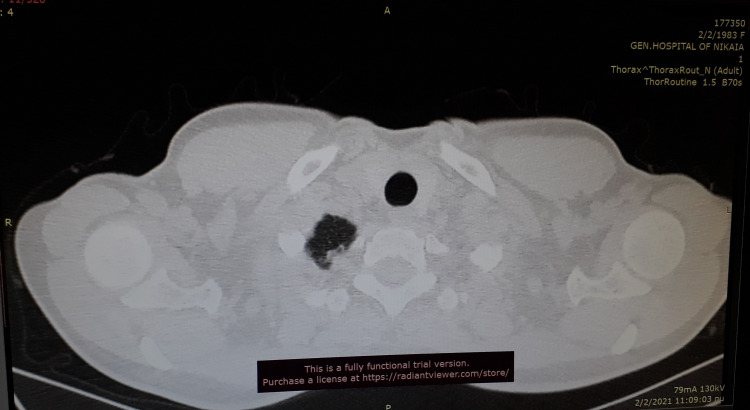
The chest CT scan showing small apical blebs at the right superior lobe
